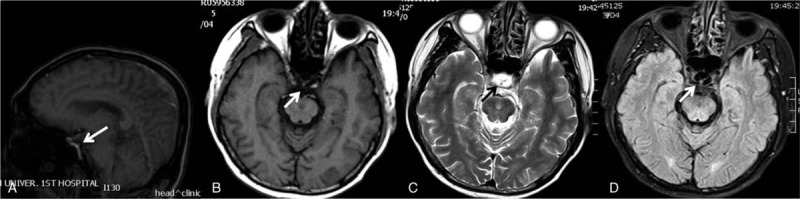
The MRI scan of the brain. (A) Sagittal T1-weighted image to show an empty sella partially filled with cerebrospinal fluid (CSF) (white arrow). (B) T1-weighted image taken in the coronal plane showing hypointensity over the sella region (white arrow). (C) T2-weighted image taken in the coronal plane showing hyperintensity with “black target sign” over the sella region (black arrow). (D) T2-tirm dark fluid image taken in the coronal plane showing hypointensity over the sella region, indicating it is filled with CSF (white arrow).
